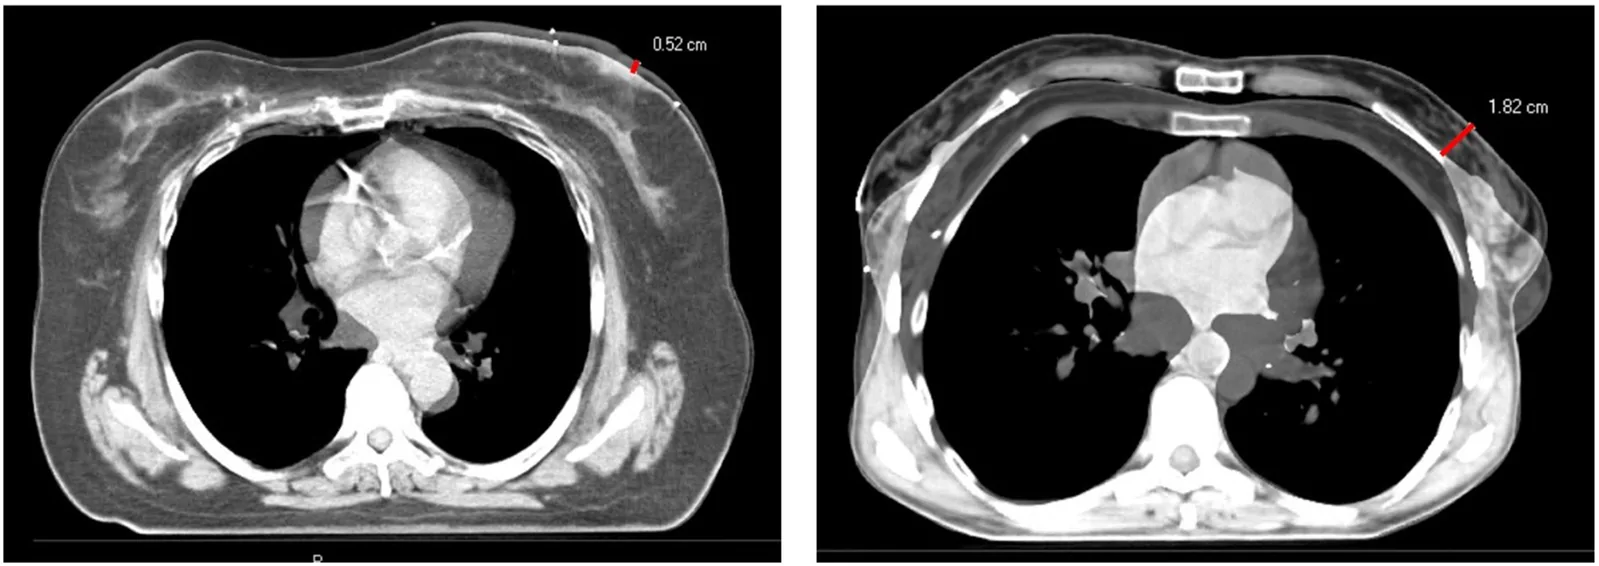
Representative example of the distance measurement between free breathing (FB) CT and deep inspiration breath hold (DIBH) CT
